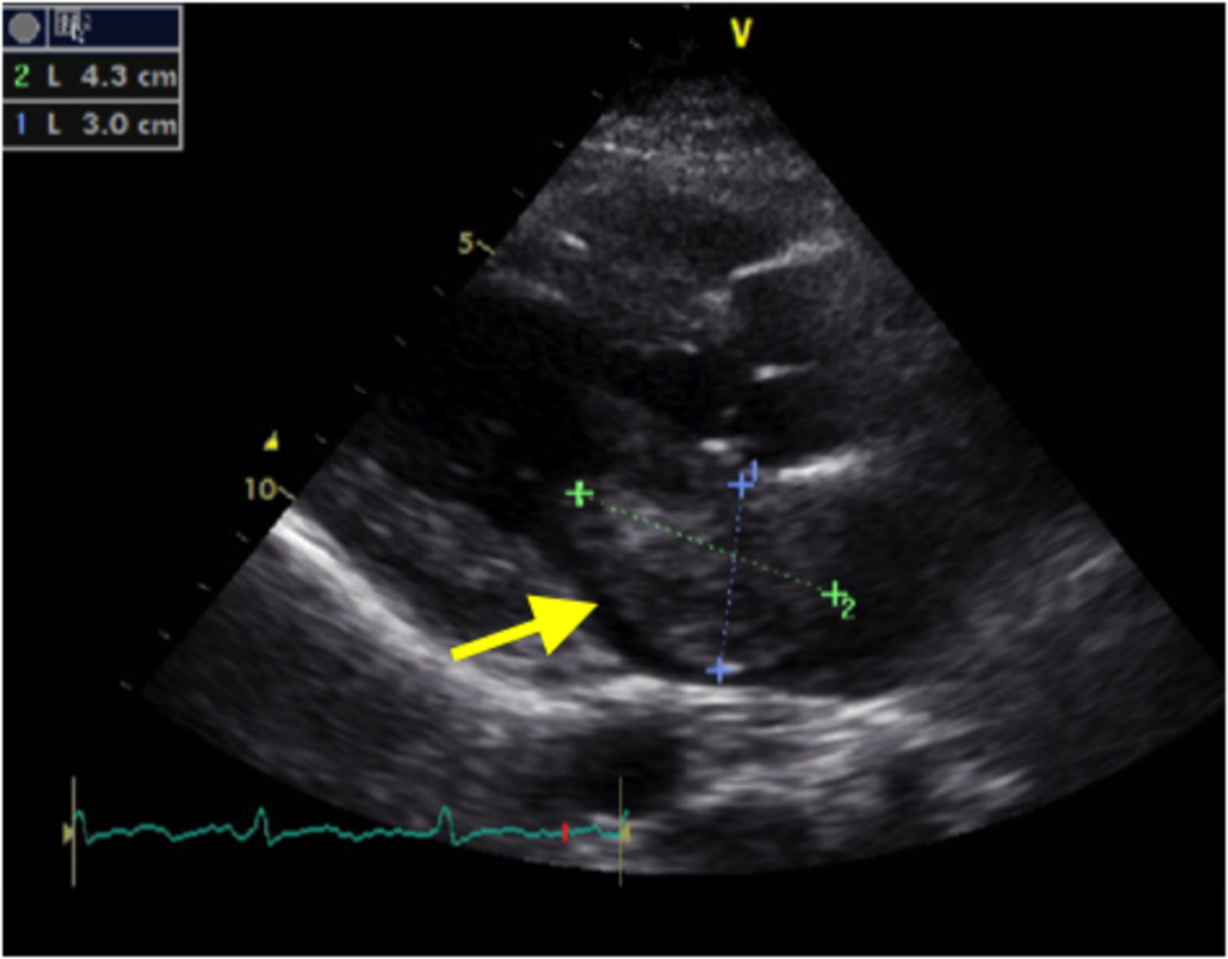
Transthoracic echocardiogram Yellow arrow pointing towards left atrial mass
